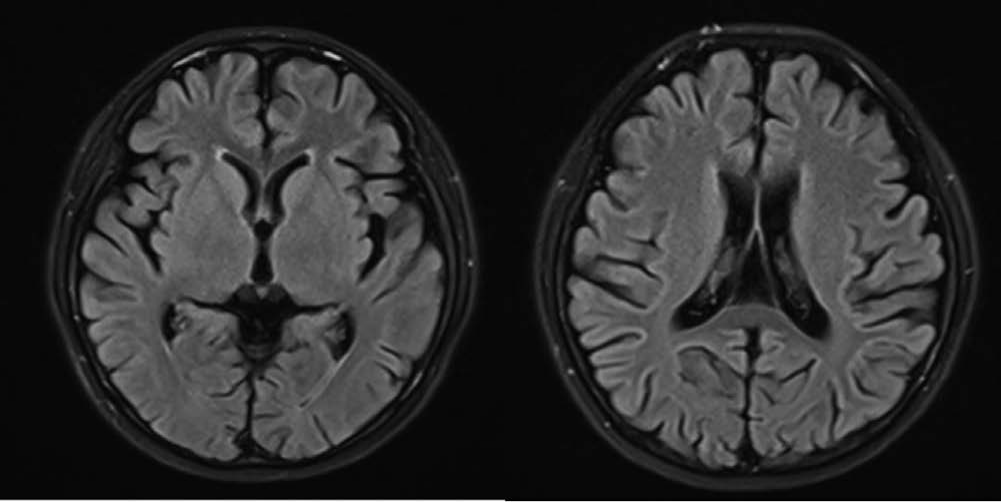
Brain MRI (2016): Mild brain atrophy. MRI = magnetic resonance imaging.
